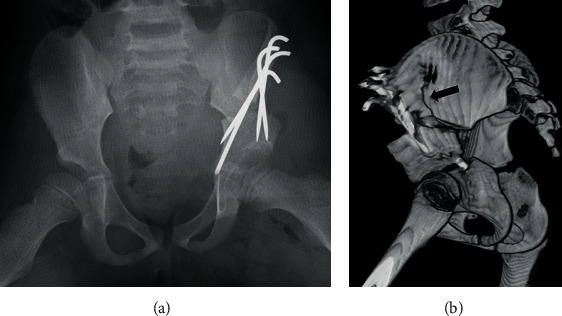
(a) The radiograph at two days after Salter innominate osteotomy. (b) 3D CT image indicating iliac fracture between the osteotomy site and iliac crest (black arrow).
